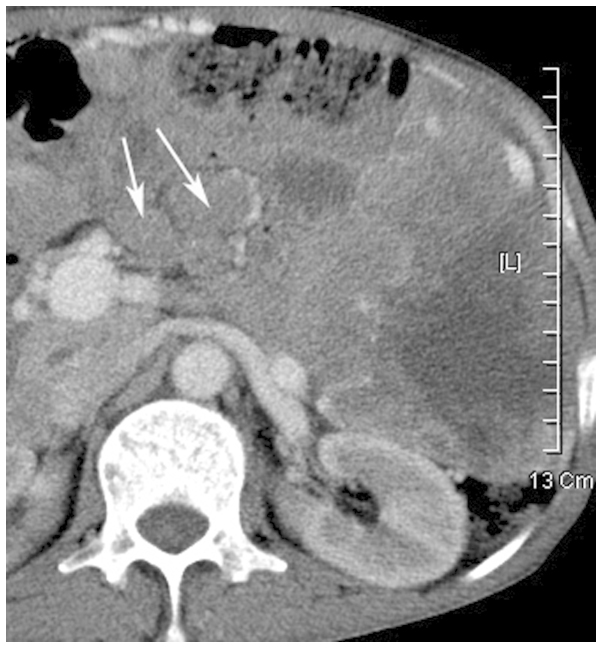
A 64-year-old male with a high-grade extragastrointestinal stromal tumor in the mesentery. Axial contrast-enhanced computed tomography image showing a 25.1-cm, irregular and ill-defind mass with heterogeneous enhancement. Enlarged mesenteric lymph nodes are also observed (arrows).
